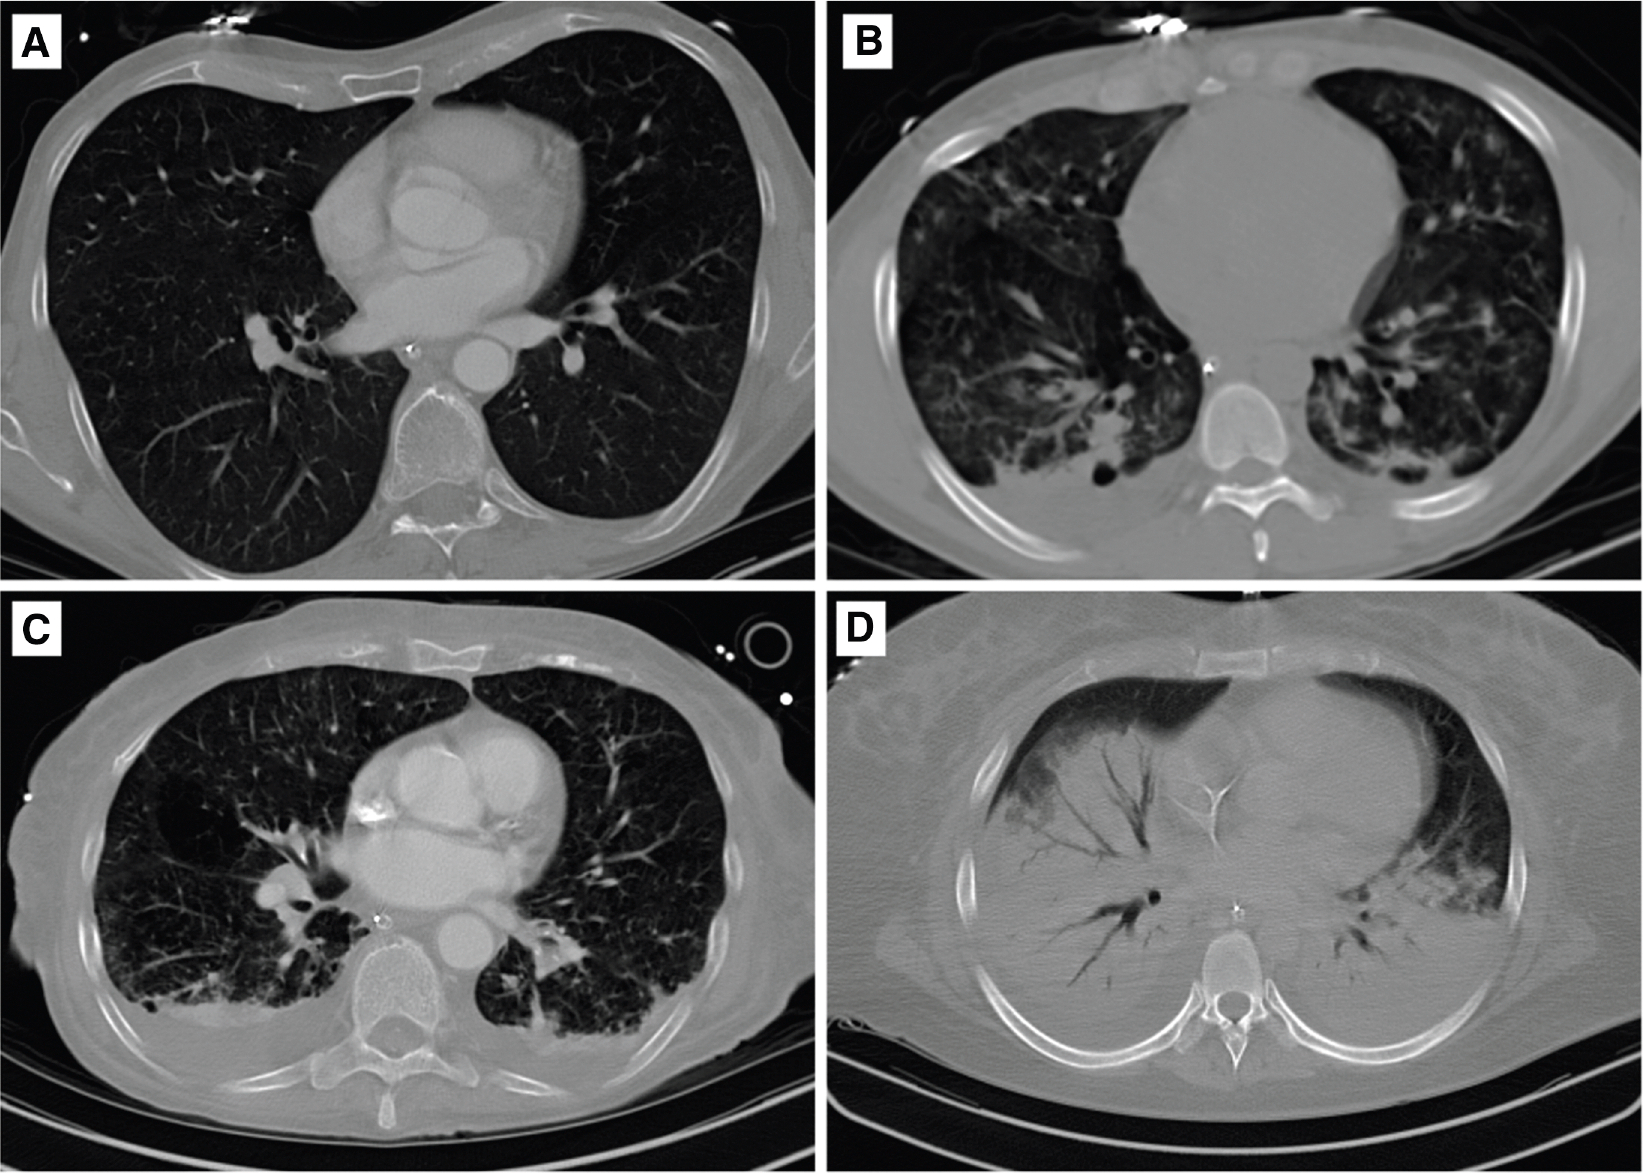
Representative computed tomography images from potential lung donors. A, No apparent abnormalities with lungs that were recovered. B, Interstitial lung disease with lungs not recovered. C, Emphysema with lungs not recovered. D, Traumatic lung contusions with lungs not recovered.
